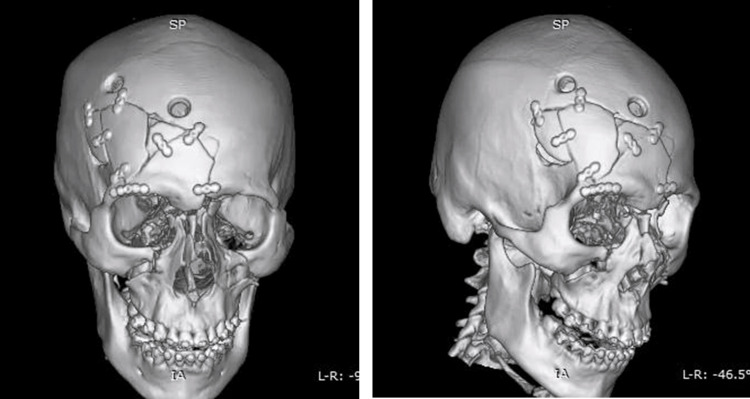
Postoperative three-dimensional CT reconstruction following the first surgical stage Left: frontal view; Right: oblique view. Findings demonstrate postsurgical changes after a bicoronal approach, right orbitozygomatic craniotomy, sequestrectomy, evacuation of epidural and subdural hematomas, frontal sinus cranialization with muscle interposition, duraplasty using pericranium, and reconstruction of the right anterior frontoparietal cranial fossa, with orbital fixation using miniplates and screws. CT: computed tomography
